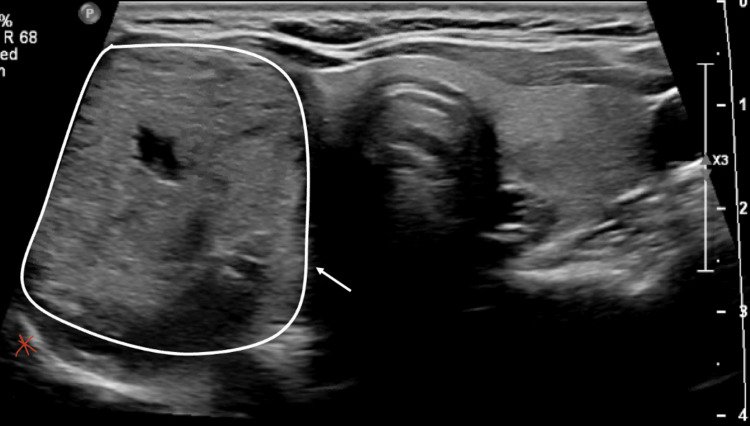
Ultrasound of the thyroid gland Ultrasound of the thyroid gland with thyroid nodule (white circle and arrow) with the typical location of the sympathetic chain indicated by red asterisk
